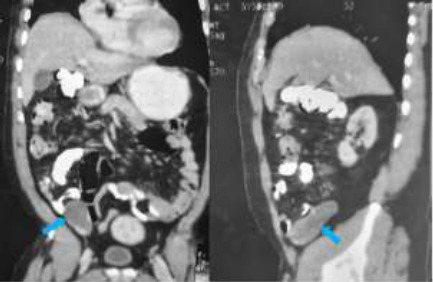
Contrast coronal, and sagittal reformatted CT images showing hypodense tubular dilatation of the appendix (blue arrows). No discernable enhancing focus within the dilated appendix
